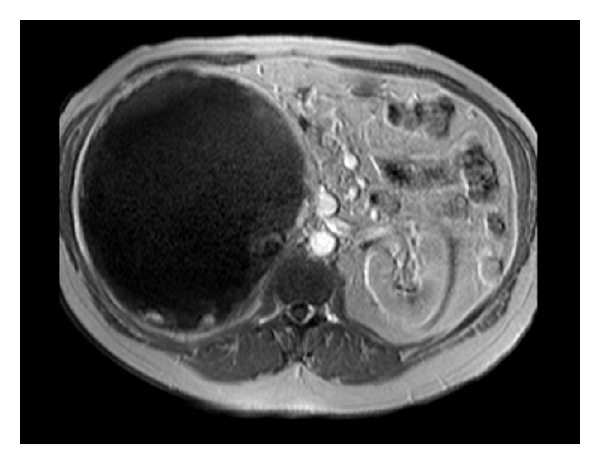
Patient's MRI T1-weighted image with characteristic high-intensity signal from the mass.
